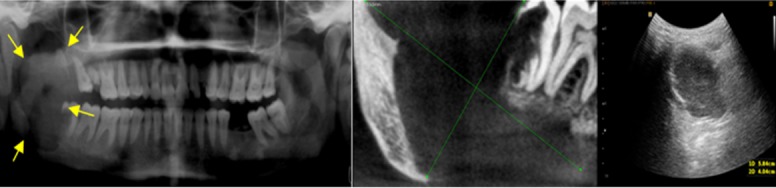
A case of ameloblastoma. (A) Panoramic view reveals large radiolucent lesion in right mandibular ramus. (B) CBCT shows large lytic lesion in right ramus. (C) Ultrasound image shows a hyperechoic lesion.
